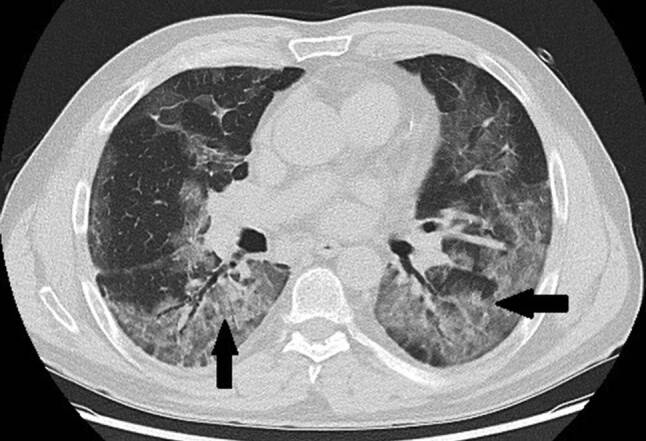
Nach 24 h nichtinvasiver Beatmungsversuche war wegen rasch progredienter, respiratorischer Verschlechterung die Intubation erforderlich. Durch Bauchlagerung und Optimierung der Beatmungsparameter gelang eine vorübergehende Stabilisation der Oxygenierung mit Oxygenationsindizes (OI) bis 150. Am Tag 13 fiel ein rasch progredientes Weichteilemphysem an Hals und linkem Hemithorax auf. Das Thorax-CT zeigte multiple Milchglastrübungen („ground-glass opacity“), Parenchymkonsolidierungen sowie einen schmalen Pneumothorax links mit einer apikalen Spaltbreite bis maximal 15 mm. Zusätzlich bestanden ein ausgedehntes Pneumomediastinum und bilaterales Weichteilemphysem am Thorax (Abb. 2). Nach Anlage einer linksseitigen Thoraxdrainage war das Weichteilemphysem zunächst rückläufig. Wegen dessen erneuter, rascher Zunahme wurden rechts und links je eine weitere Thoraxdrainage gelegt, obwohl rechts kein Pneumothorax nachweisbar war. Danach war das Weichteilemphysem kontinuierlich regredient, die Lungen blieben expandiert. Trotzdem verschlechterte sich die respiratorische Situation unaufhaltsam. Der Patient verstarb nach 17 Tagen invasiver Beatmung (Pressure-Support-Ventilation-Modus, Spitzendrücke 28 mbar, durchschnittlicher „driving pressure“ 17 mbar) und begleitender, intensivmedizinischer Maßnahmen infolge kardiorespiratorischen Versagens.
